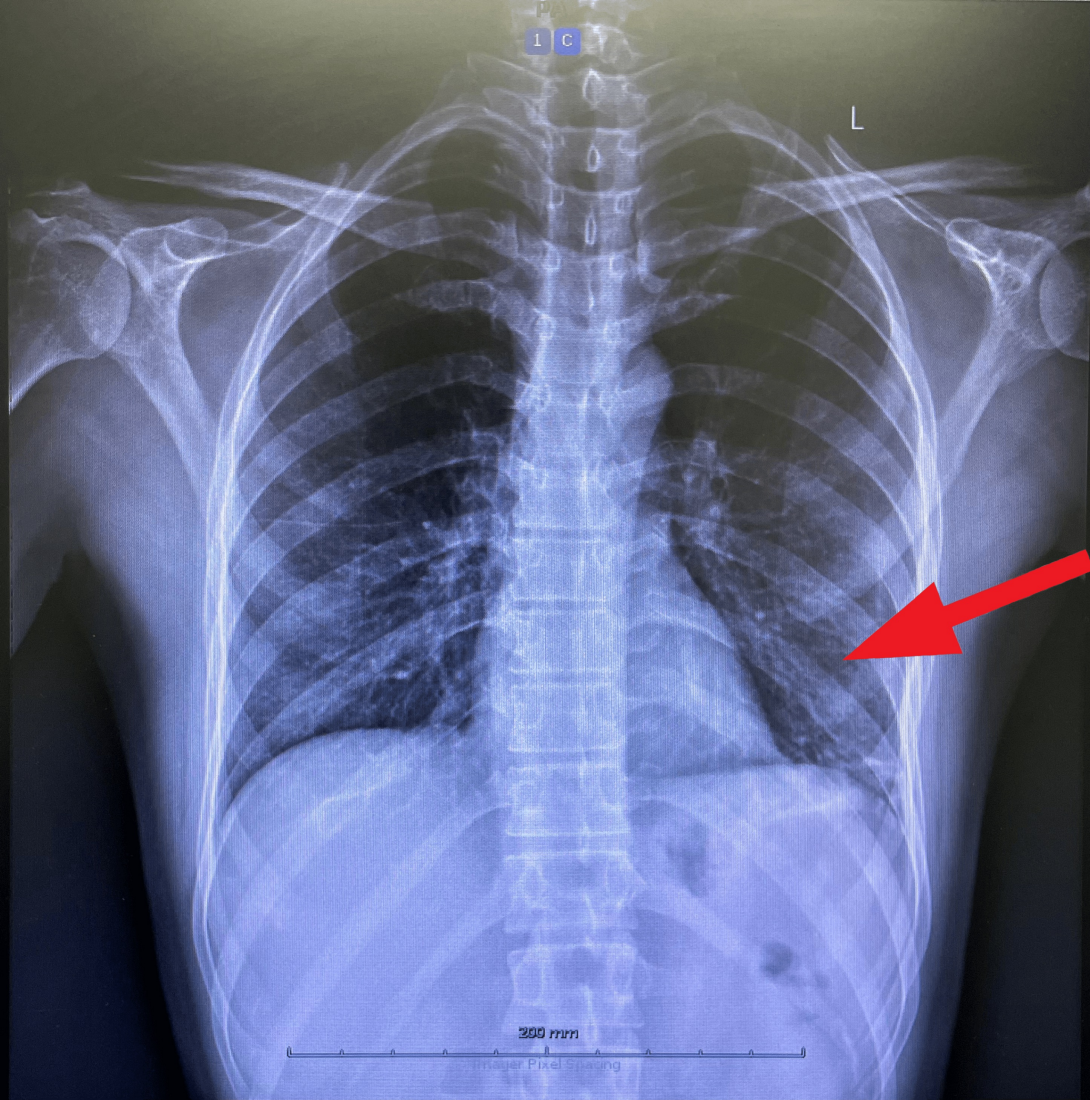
Patient's chest X-ray Atelectatic changes were noted in the left lung base (red arrow).
